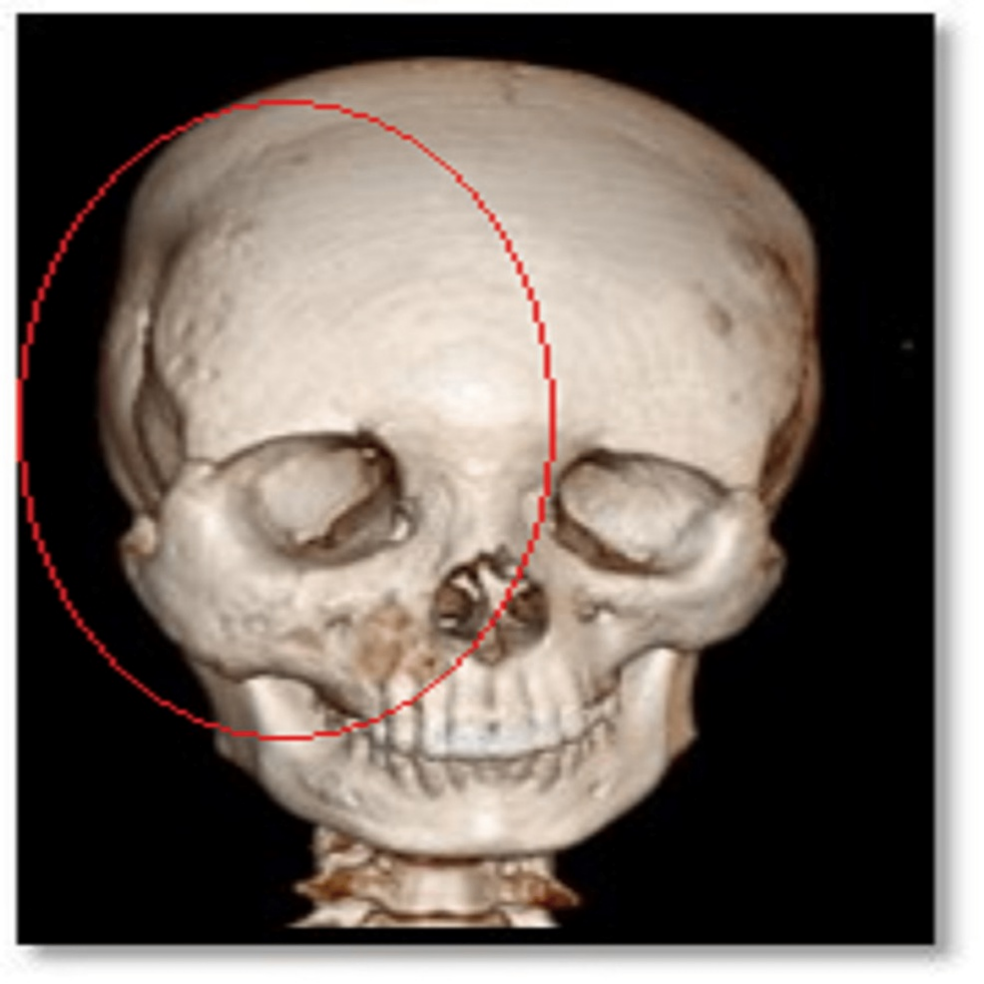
CECT head (3D cut) showing bony expansion over the right craniofacial region CECT: contrast-enhanced computed tomography
